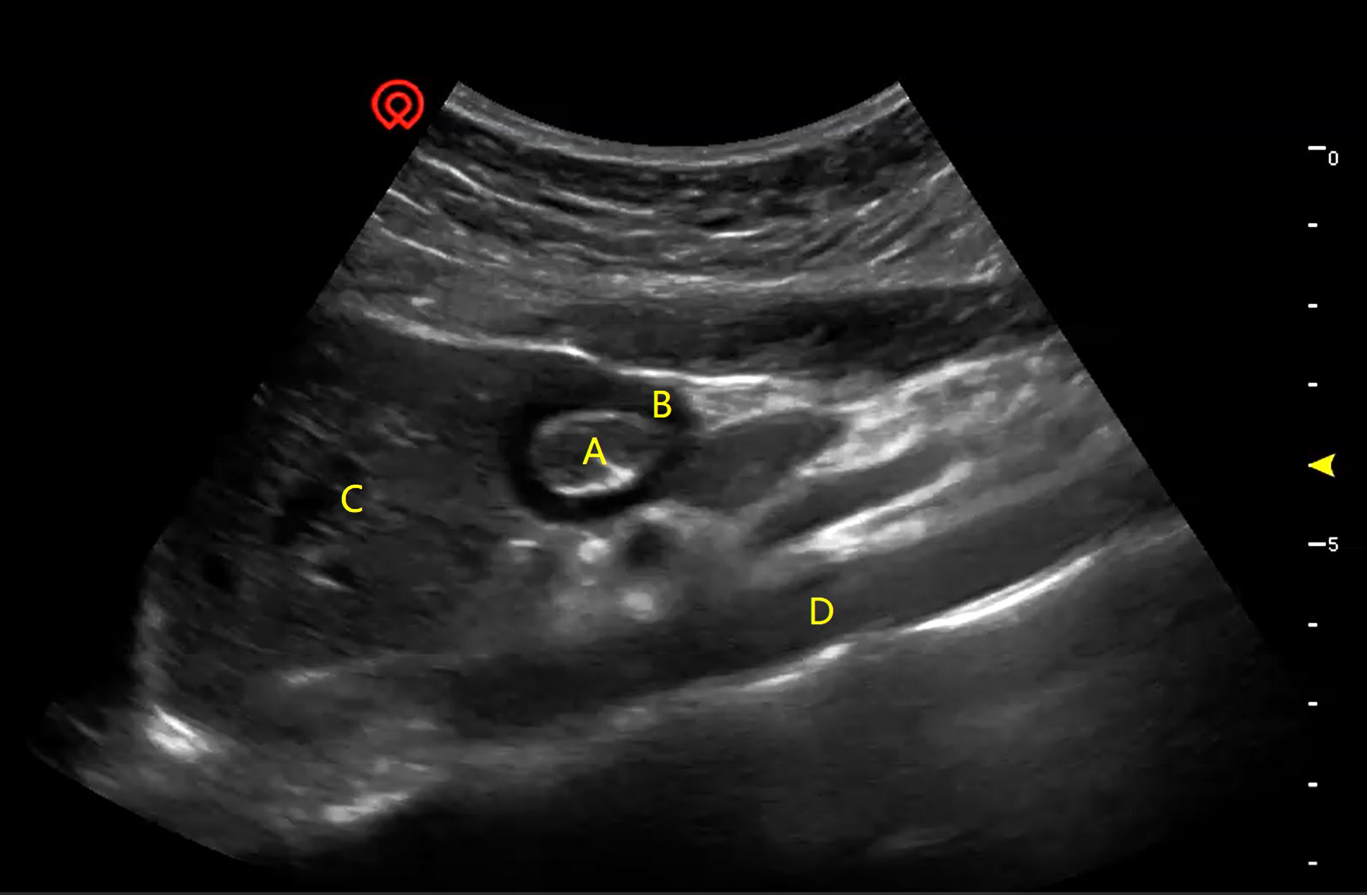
A typical image of preoperative ultrasonography of the gastric antrum and surrounding structures. (A) CSA-ISMP, the cross-sectional area of the inner side of the muscularis propria; (B) TMP, the thickness of the muscularis propria; (C) the left hepatic lobe; (D) the aorta.
